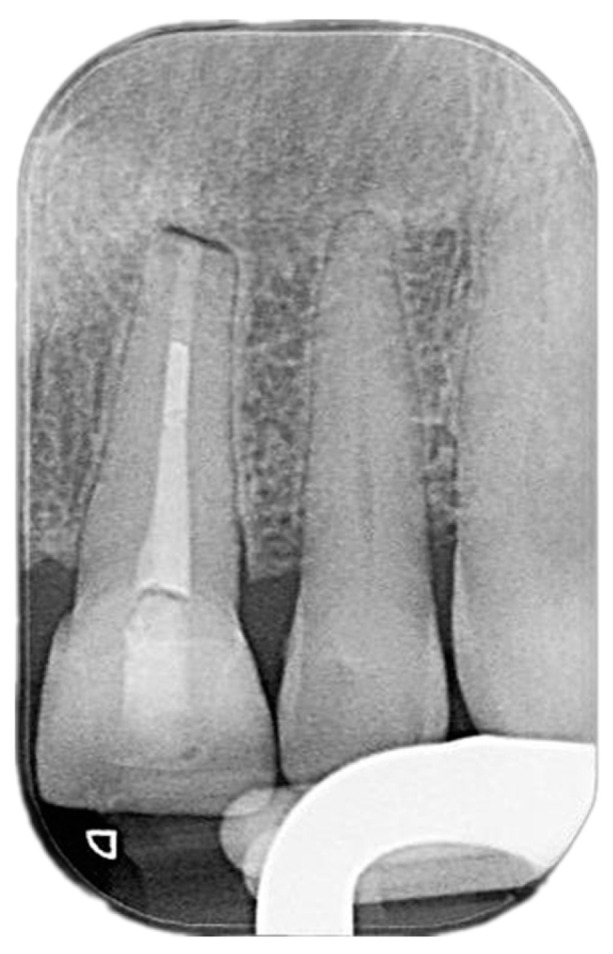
Two-and-a-half-year-review (30 months) post-surgery intraoral periapical radiograph of upper left central and lateral incisors.
